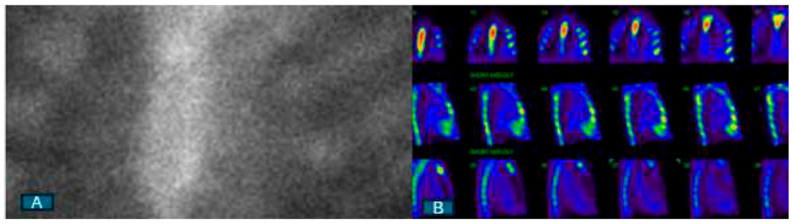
Negative 99mTc-labeled nuclear scintigraphy. (A) Visual grade 0 on planar image; (B) absence of myocardial tracer uptake on single-photon emission computed tomography.
